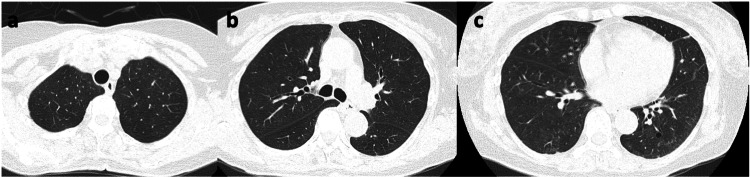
Chest CT images of the patient before discharge. (a-c) Chest CT scans before discharge revealed improved ground-glass opacities and consolidation observed on admission
